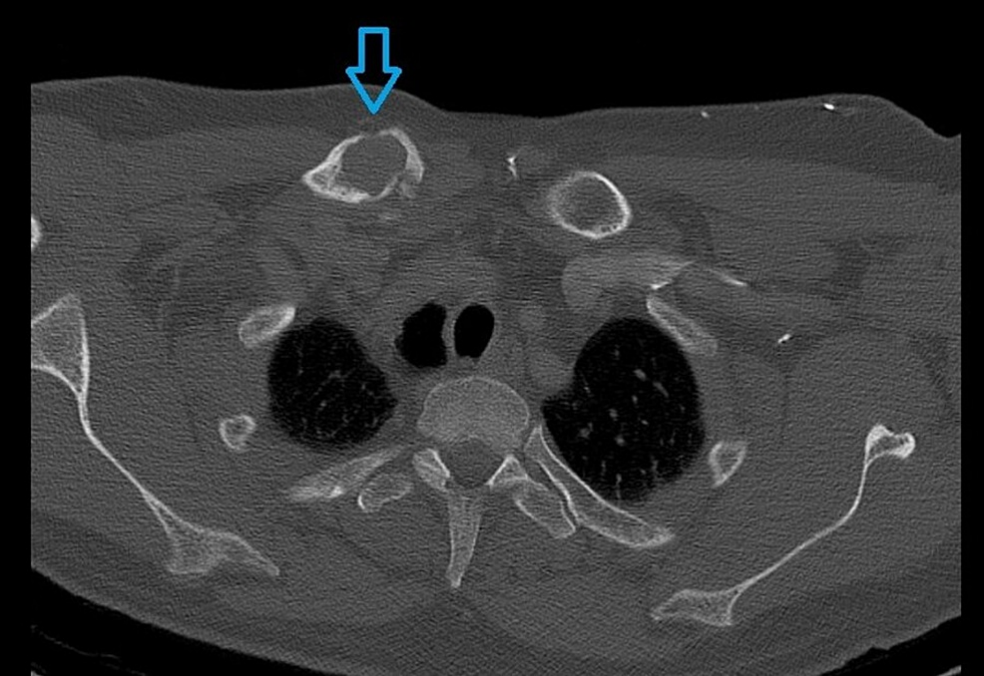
A CT scan showing an osteolytic damage to the clavicle (blue arrow).
